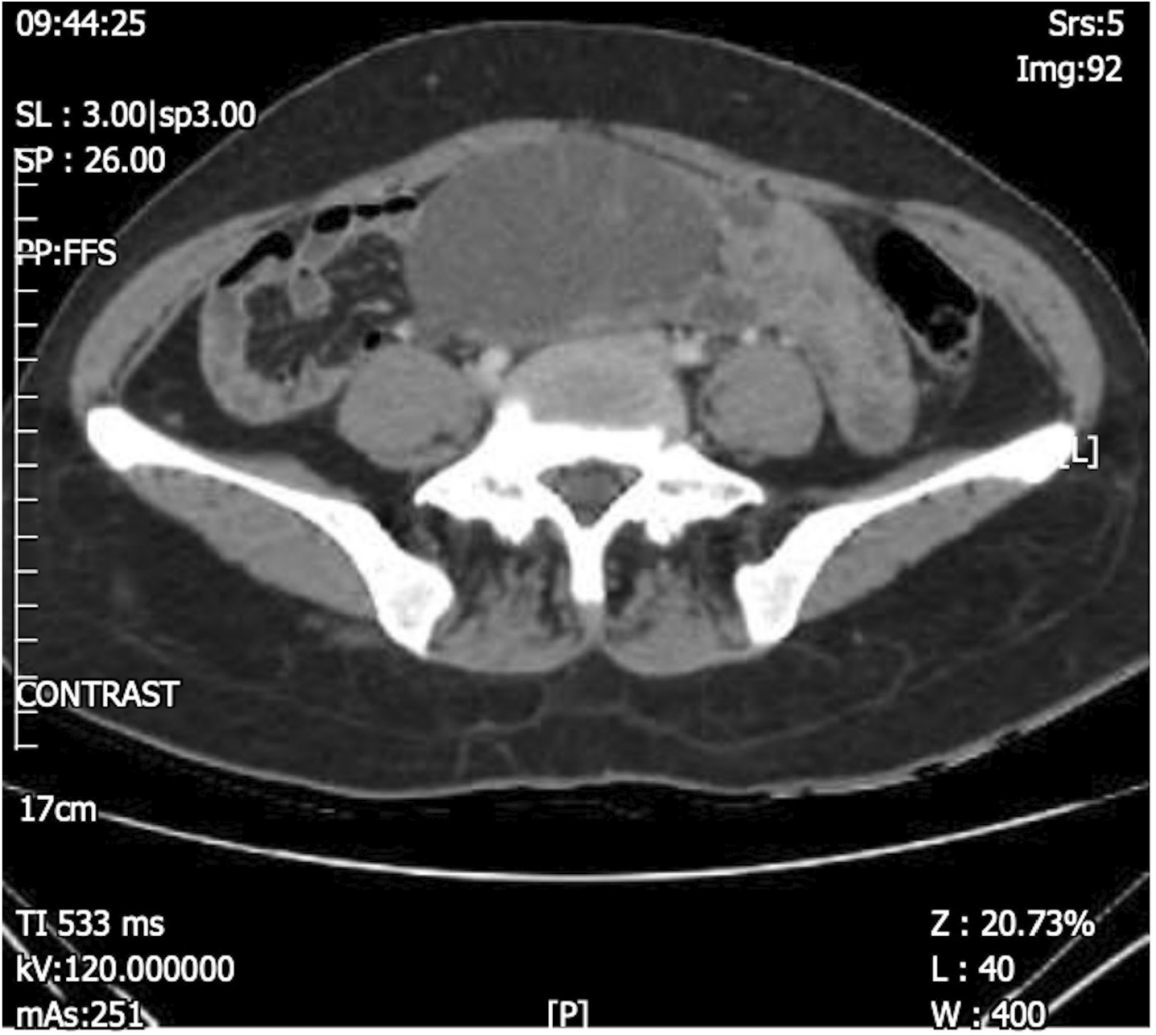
Abdominopelvic computed tomography (CT), axial, with intravenous and oral contrast, revealed a large cystic mass arising from the left adnexa with several thin enhancing septa highly suggestive of ovarian cystadenocarcinoma.
